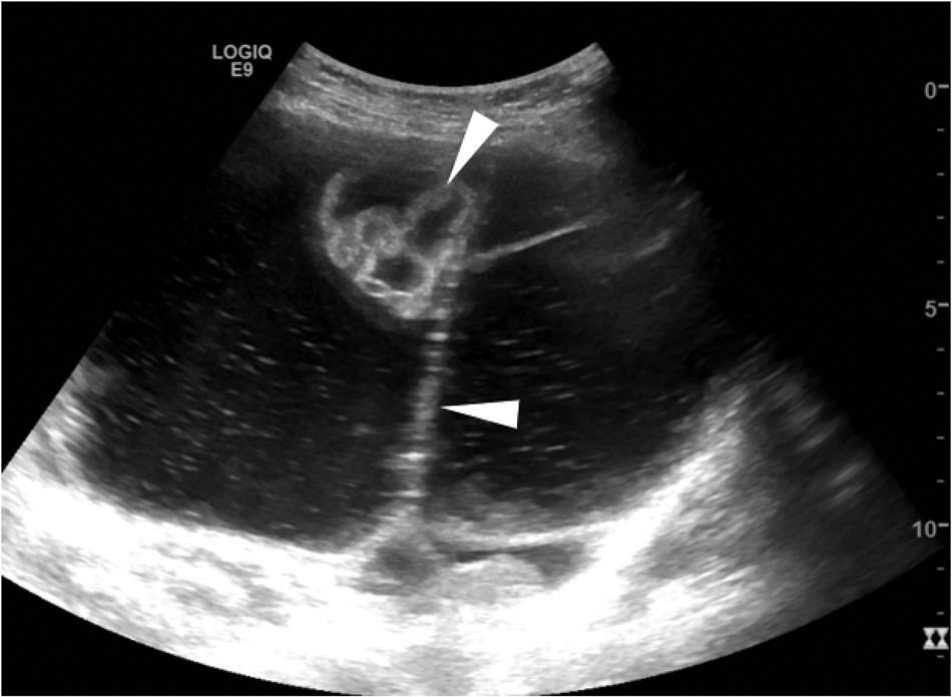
Ultrasound: Transabdominal pelvic ultrasound demonstrates an approximately 19 cm complex cystic mass occupying the lower abdomen and pelvis. There are internal solid components and septations (arrowheads), findings that are concerning for neoplasm.
